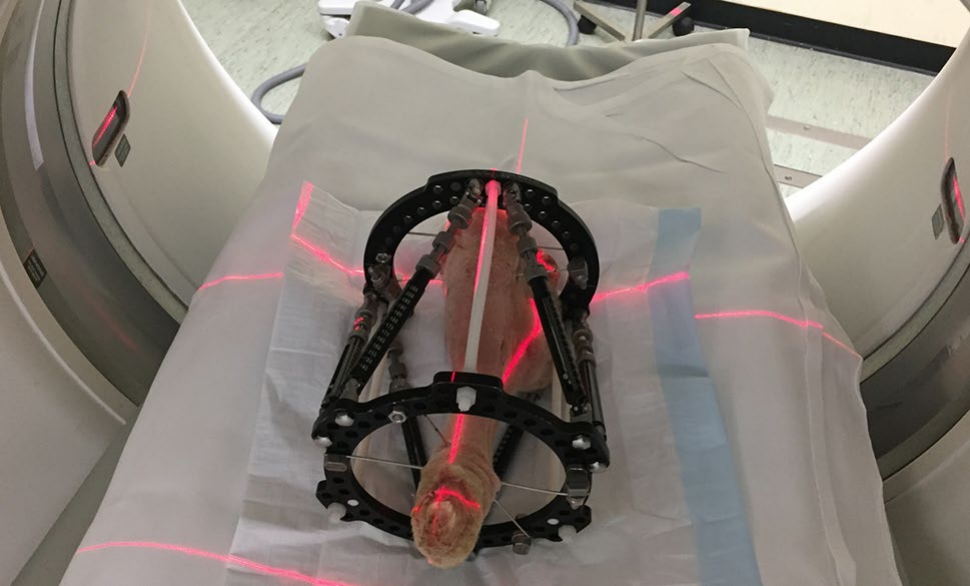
Photograph of the frame/leg construct in the CT scanner
